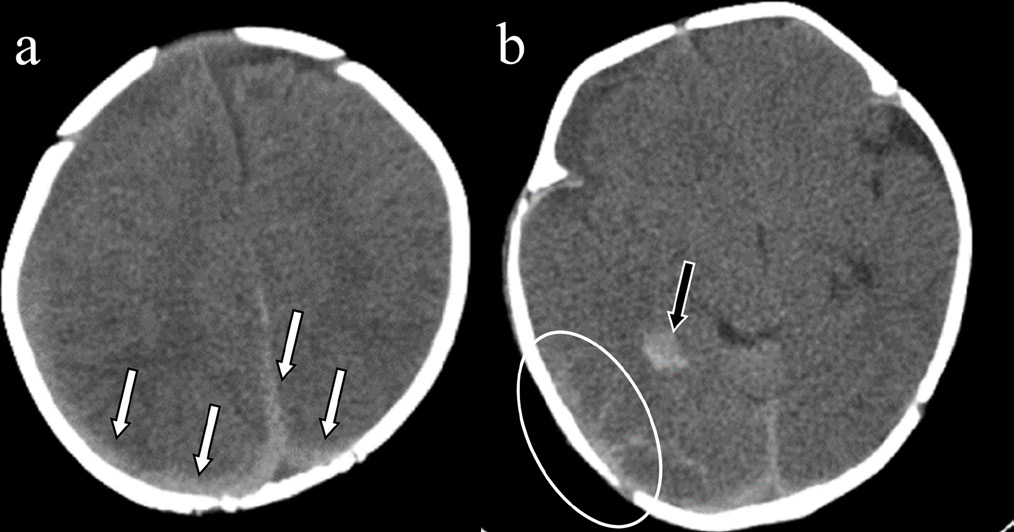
Intracranial haemorrhage caused by vitamin K deficiency. A 2-month-old boy presented with disturbance of consciousness and decreased feeding. Head computed tomography demonstrated subdural haematoma around the falx and posterior area (a; white arrows), subarachnoid haemorrhage on the surface of the right temporal lobe (b; circle) and intraparenchymal haemorrhage in the temporal lobe (b; black arrow). He was examined the causes of bleeding tendency and diagnosed with biliary atresia.
